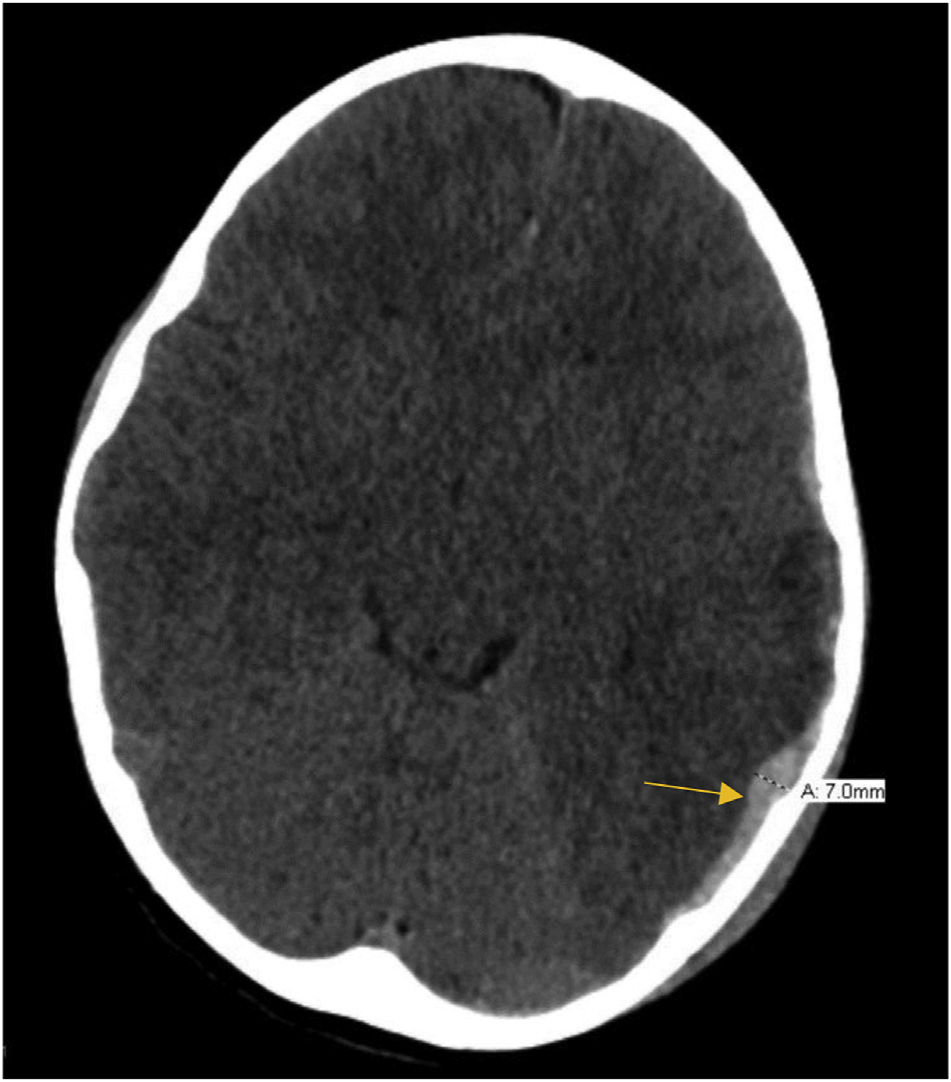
CT scan taken on second day of hospital admission after onset of bradycardic episodes. Results indicated expanding hematoma and development of midline shift.
